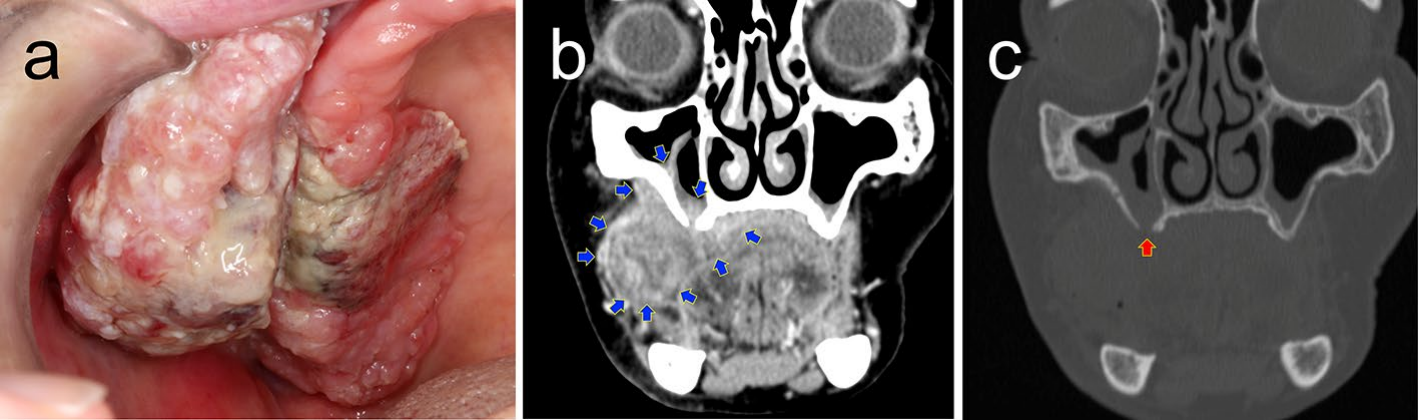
Clinical presentation. a Intraoral finding. Representative coronal computed tomography (CT) images with bone window (b) and with contrast-enhanced (c). The blue arrows indicate a large tumor extension (b). The red arrow indicates a bone penetration at the alveolar process of the right maxilla (c)
